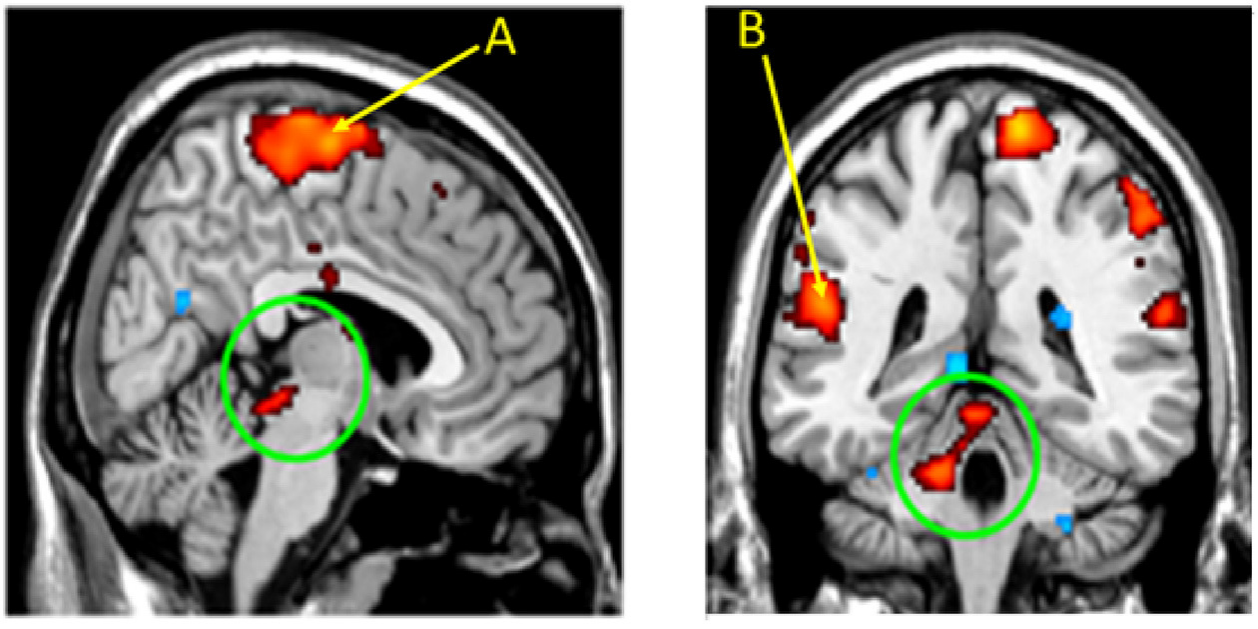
Cerebellar and parabrachial pontine nuclei are activated by limb proprioceptive sensory stimulation. Functional MRI images following passive right foot movement in 14 children activated the parabrachial pons (Left circled area) and medial cerebellum (right circled area), sites involved in respiratory timing and blood pressure control. The sensorimotor cortex for the foot (A) and for the cervical region (for diaphragm), (B) are also activated, indicating that locomotion (foot) and breathing motor sites are concurrently recruited by the proprioceptive stimuli. From (51).
